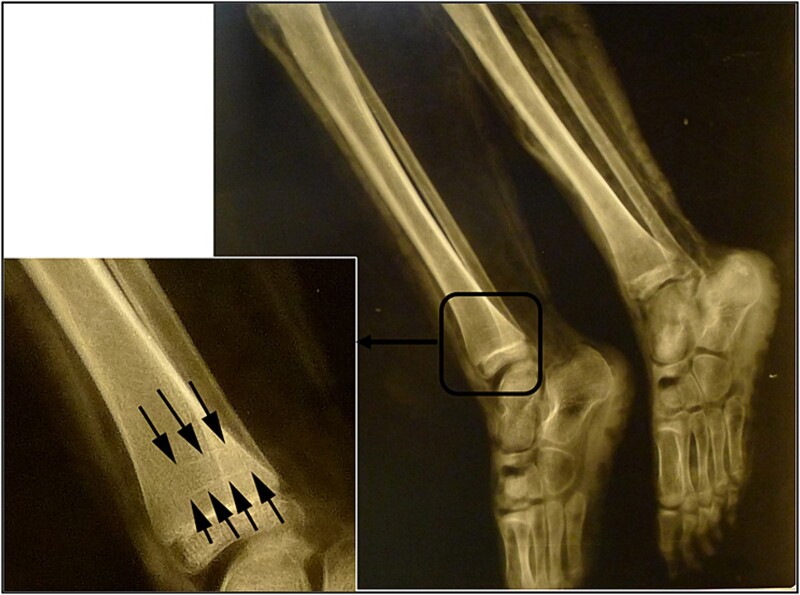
Radiograph showing the Harris lines on the distal metaphysis of the right tibia.
